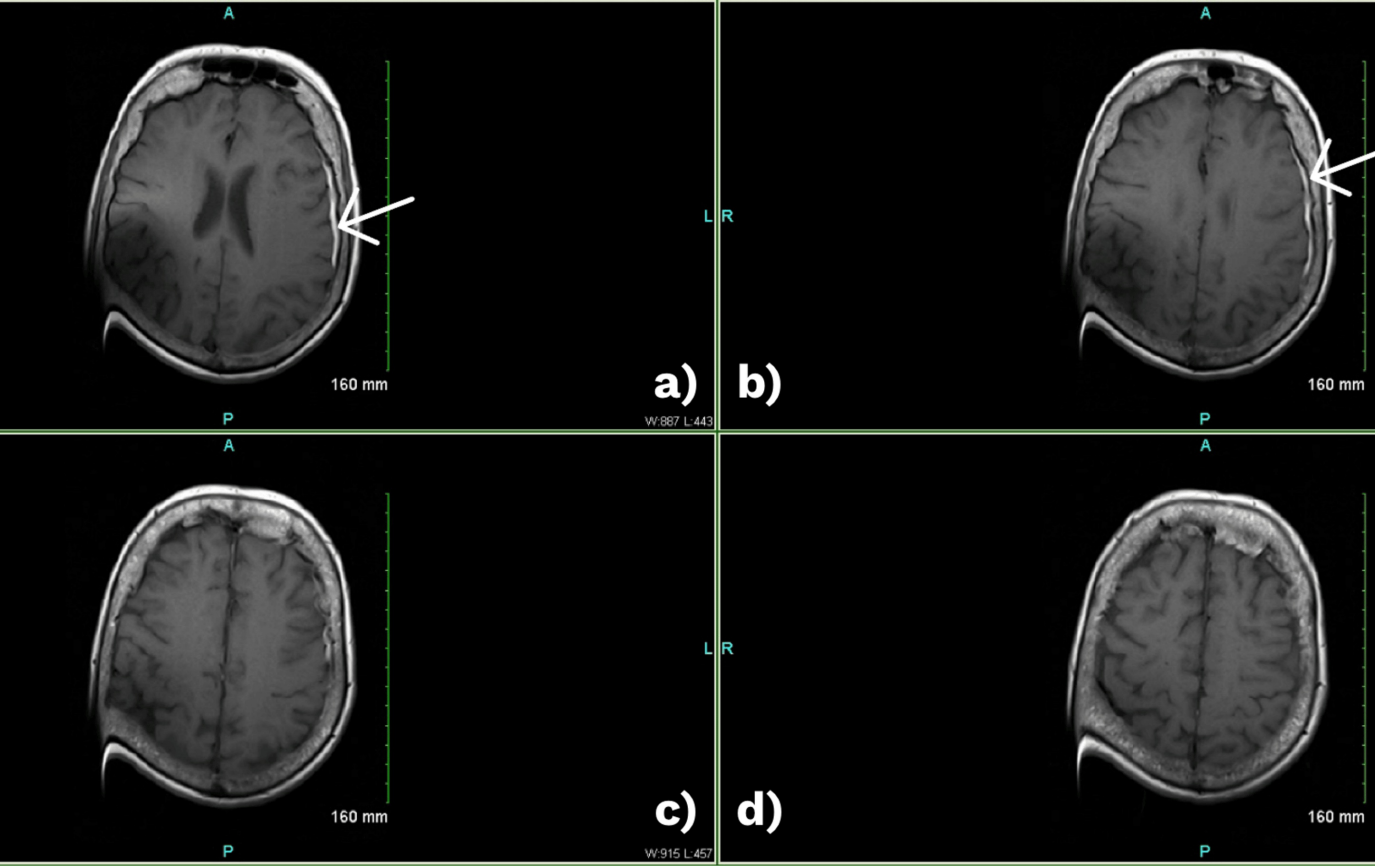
Initial MRI brain (axial T1-weighted image) a, b: subtle crescentic extra-axial hypointensity (white arrows) along the left temporoparietal convexity consistent with a small subdural hematoma (~3.1 mm); c, d: no acute infarction, mass effect, or midline shift. Image quality is limited by susceptibility artifact from the right cochlear implant.
